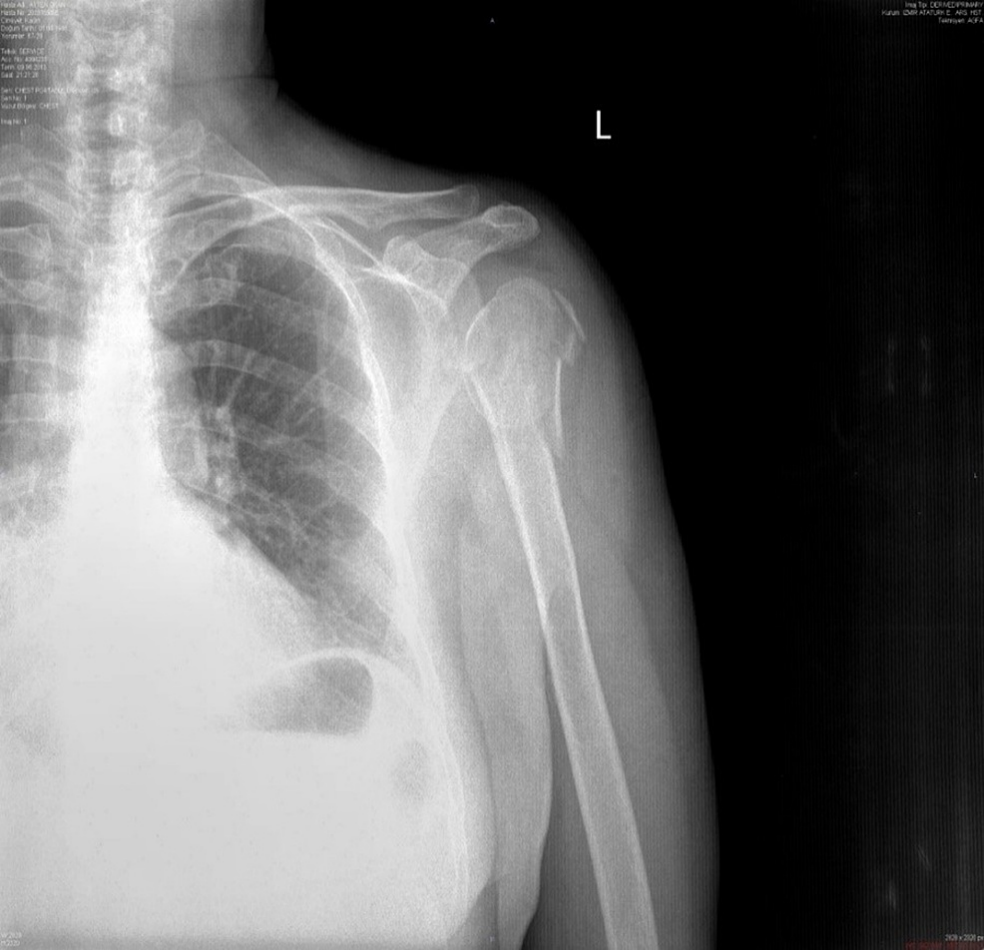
A 70-year-old female’s first X-ray.
